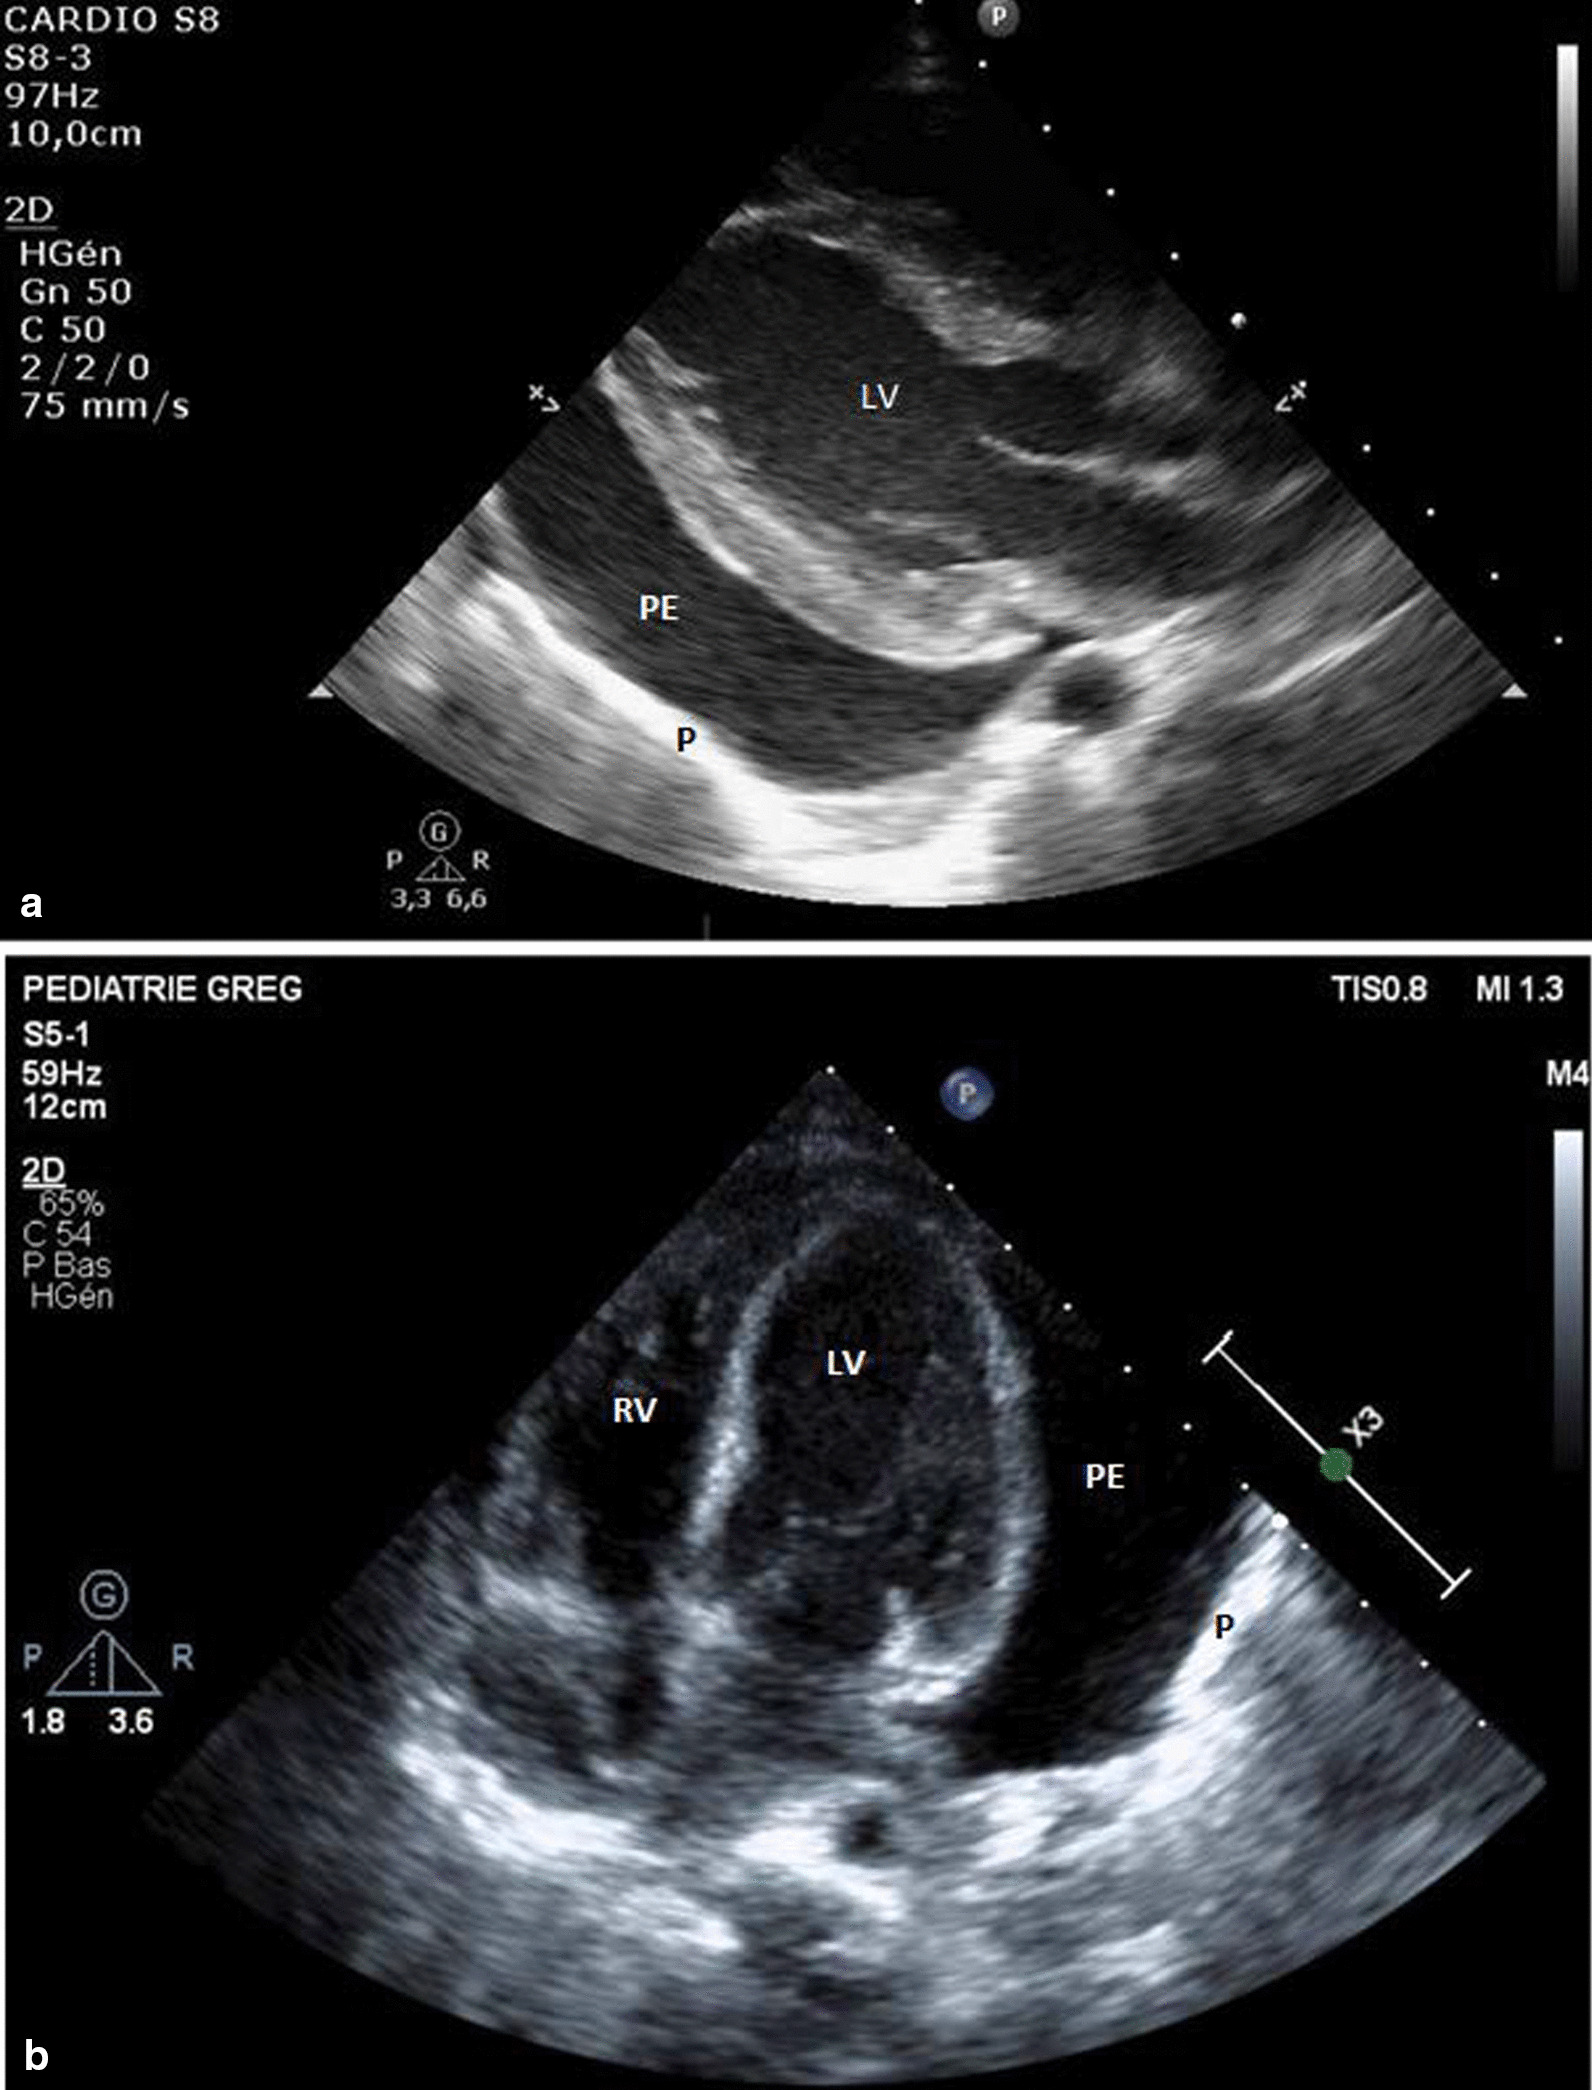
Echocardiography showing pericardial effusion predominantly surrounding the left ventricle before pericardiocentesis. a Parasternal long-axis view. b Apical four-chamber view. *P *pericardium, *PE *pericardial effusion, *RV *right ventricle, *LV *left ventricle
